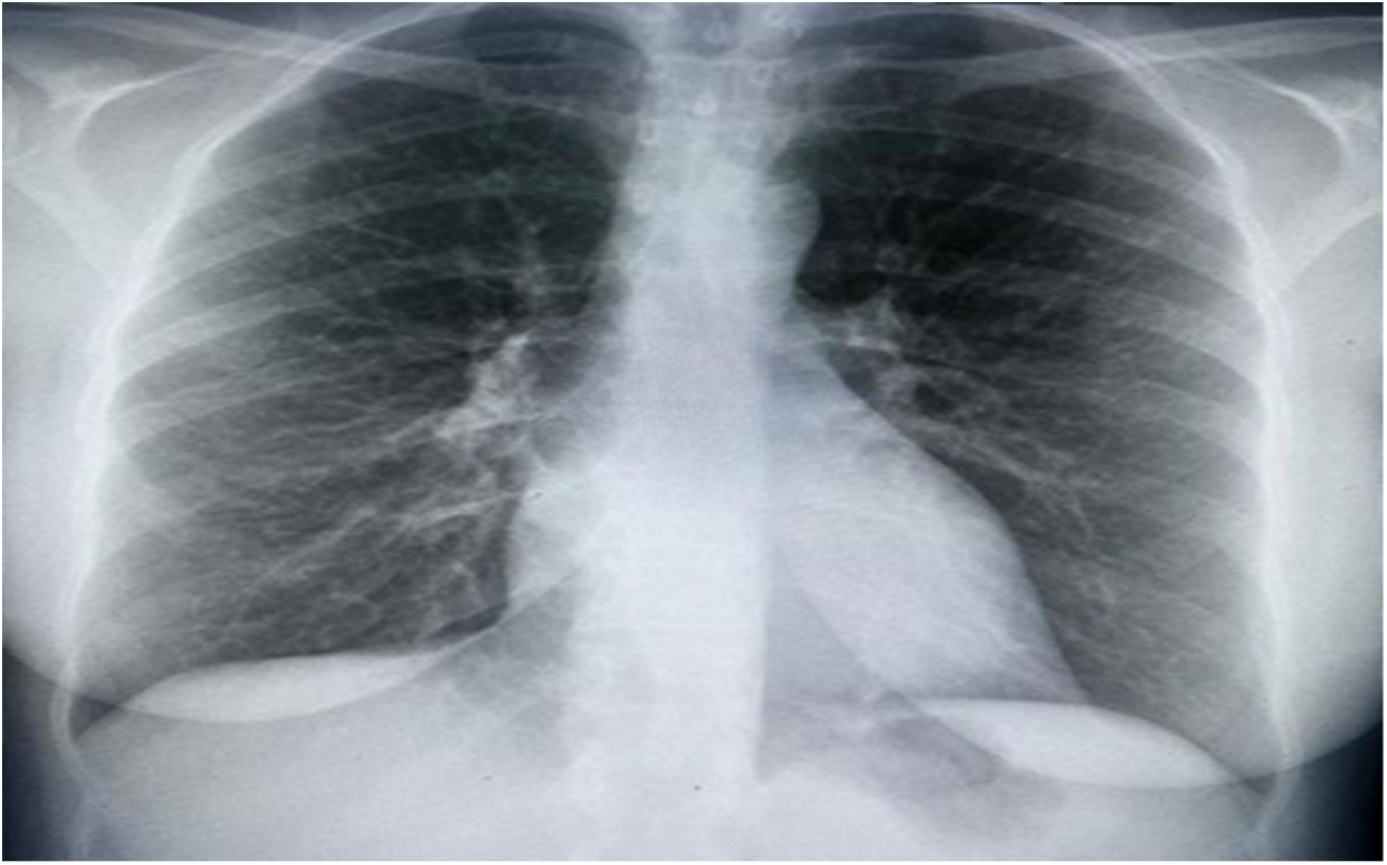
Chest x-ray showing bilateral interstitial opacities consistence with pulmonary congestion.
